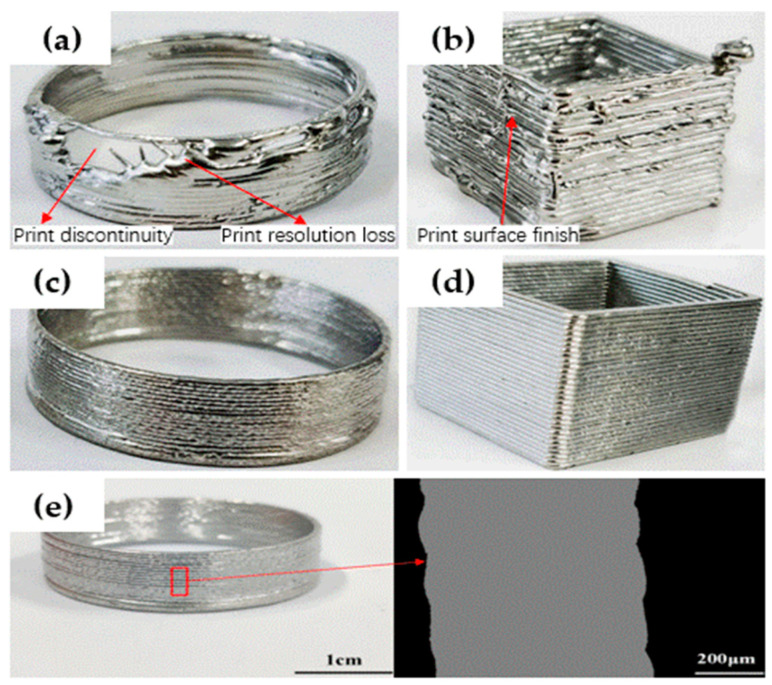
Print quality of samples using (a,b) DMW (c,d) ultrasound-assisted DMW, and (e) Interlayer bonding of the highlighted area [151].
